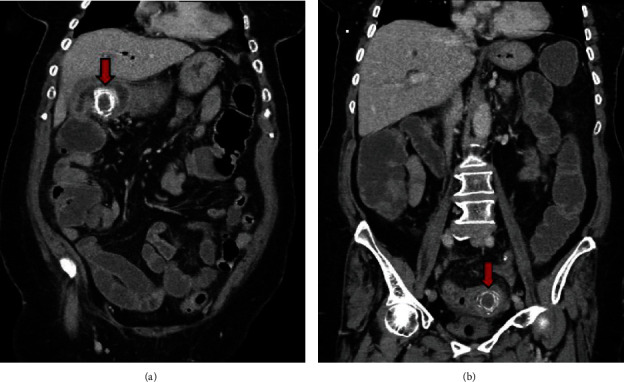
The first gallstone in the first part of the duodenum (a) and the second gallstone in the sigmoid colon (b) shown on coronal CT scan.
